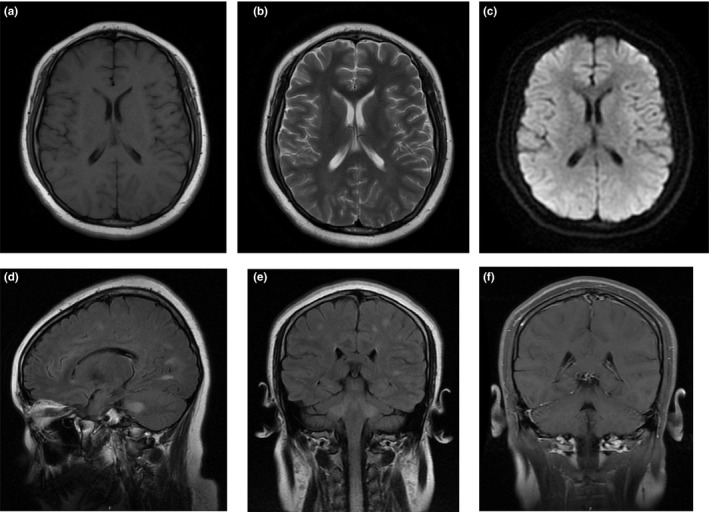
MRI‐scan 3 days after symptom onset. Brain MRI FLAIR image showing areas of hyperintensity involving subcortical white matter of both cerebral hemispheres, pedunculus cerebellaris medius bilaterally, and upper cervical cord. But not obvious on other MRI sequences. (a) Axial T1. (b) Axial T2. (c) Axial DWI (diffusion‐weighted imaging). (d) Sagittal T2‐FLAIR. (e) Coronal T2‐FLAIR. (f) Coronal T1 gadolinium‐enhanced
